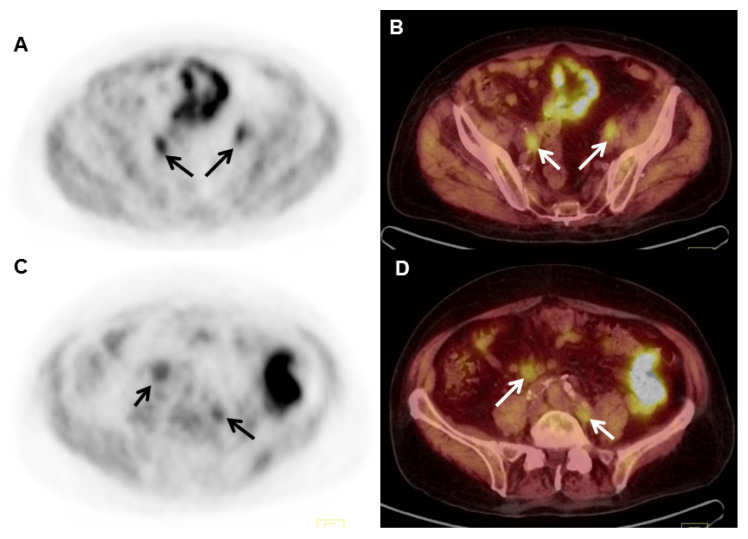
An 83-year-old man with prostate adenocarcinoma status after radical prostatectomy and biochemical recurrence (PSA = 6.3 ng/mL). Axial PET/CT (A) and axial fused PET/CT (B) images demonstrate increased ^18^F-choline activity (SUVmax = 5.4) localizing to enlarged lymph nodes in the bilateral external iliac regions, consistent with nodal metastases (arrows). Axial PET (C) and axial fused PET/CT (D) images demonstrate focal increased ^18^F-choline activity (SUVmax = 4.5) localizing to enlarged retroperitoneal lymph nodes, compatible with nodal metastases (arrows).
